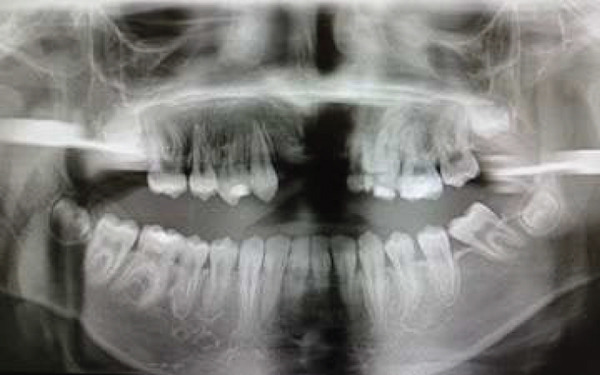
Preoperative panoramic radiograph showing multiple dental anomalies in a child with Tatton–Brown–Rahman syndrome.
